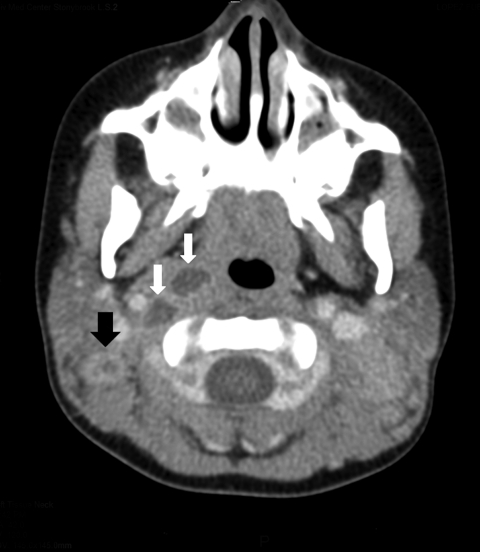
Computed tomography scan of the neck of a 3-year-old girl, showing right lateral retropharyngeal abscess (white arrows) and enlarged bilateral posterior cervical lymph nodes with low attenuation of a right cervical lymph node (black arrow), consistent with atypical mycobacterium adenitis.
